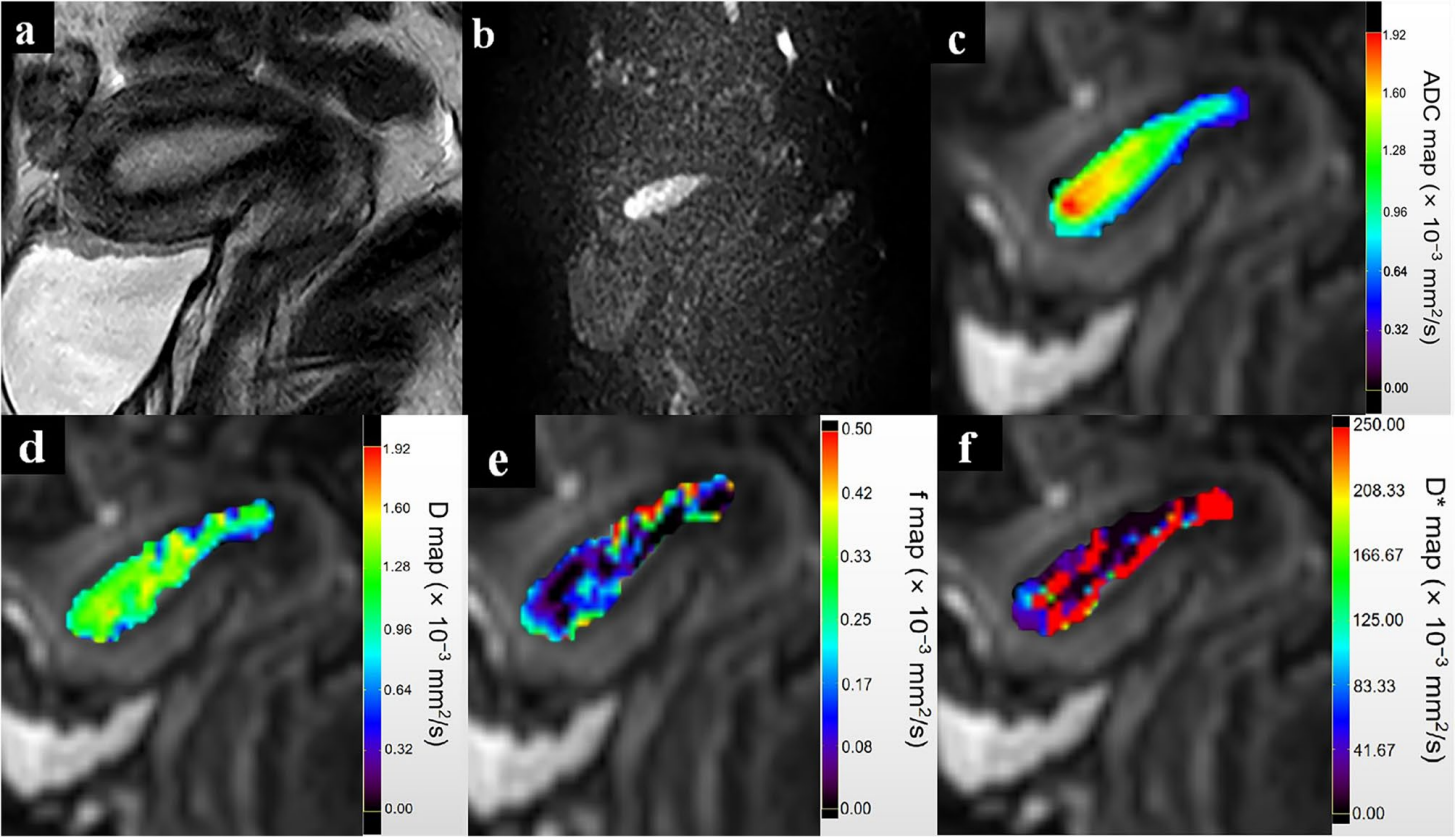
A 24-year-old healthy woman. (a) Sagittal T2-weighted turbo spin-echo (TSE) image demonstrates the long ribbon shape normal endometrium with high signal intensity. (b) Sagittal diffusion-weighted image (b = 800 s/mm^2^) of the uterus. Parametric maps of ADC (c), *D* (d), *f* (e) and *D (f) demonstrate that the average ADC, *D*, *f* and *D values of the normal endometrium are 1.51 × 10^−3^ mm^2^/s, 1.36 × 10^−3^ mm^2^/s, 0.12 and 66.82 × 10^−3^ mm^2^/s, respectively. *ADC* apparent diffusion coefficient, *D* true diffusion coefficient, *f* perfusion fraction, *D pseudodiffusion coefficient.
